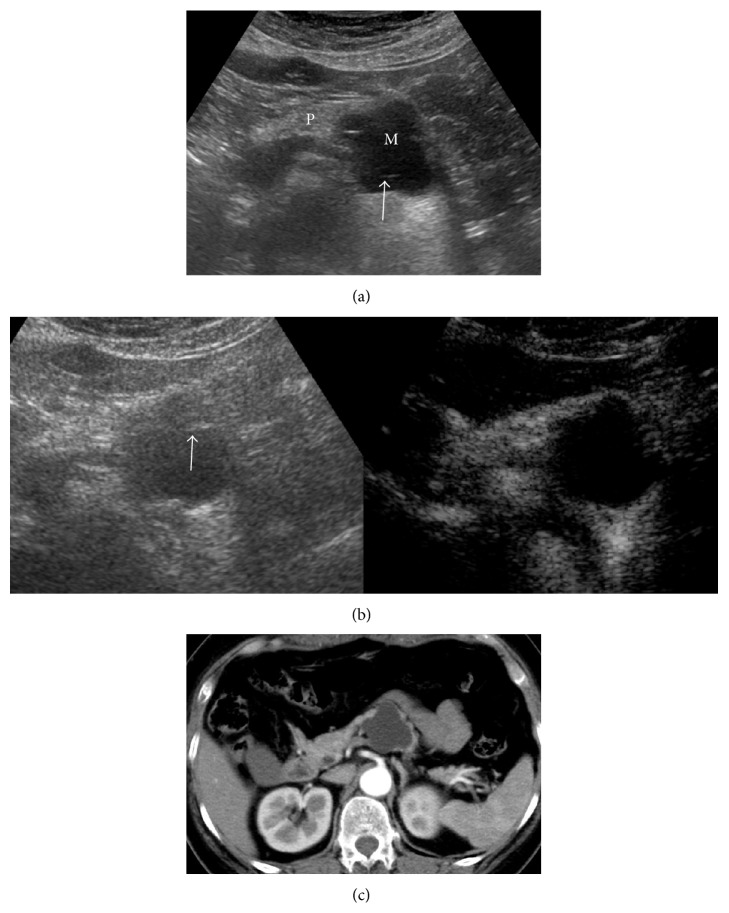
Pancreatic lesion was found by physical examination and was diagnosed as MCN by surgical pathology. (a) Cystic lesions in the tail of the pancreas were indicated by US (M: mass; P: pancreas), with multiple septa (arrow). The case was diagnosed as type III. (b) Enhancement was not shown in cystic lesions in CEUS (the right picture). The case was diagnosed as type I. (c) Enhanced CT indicated no enhancement in the cystic lesion. The case was diagnosed as type I.
